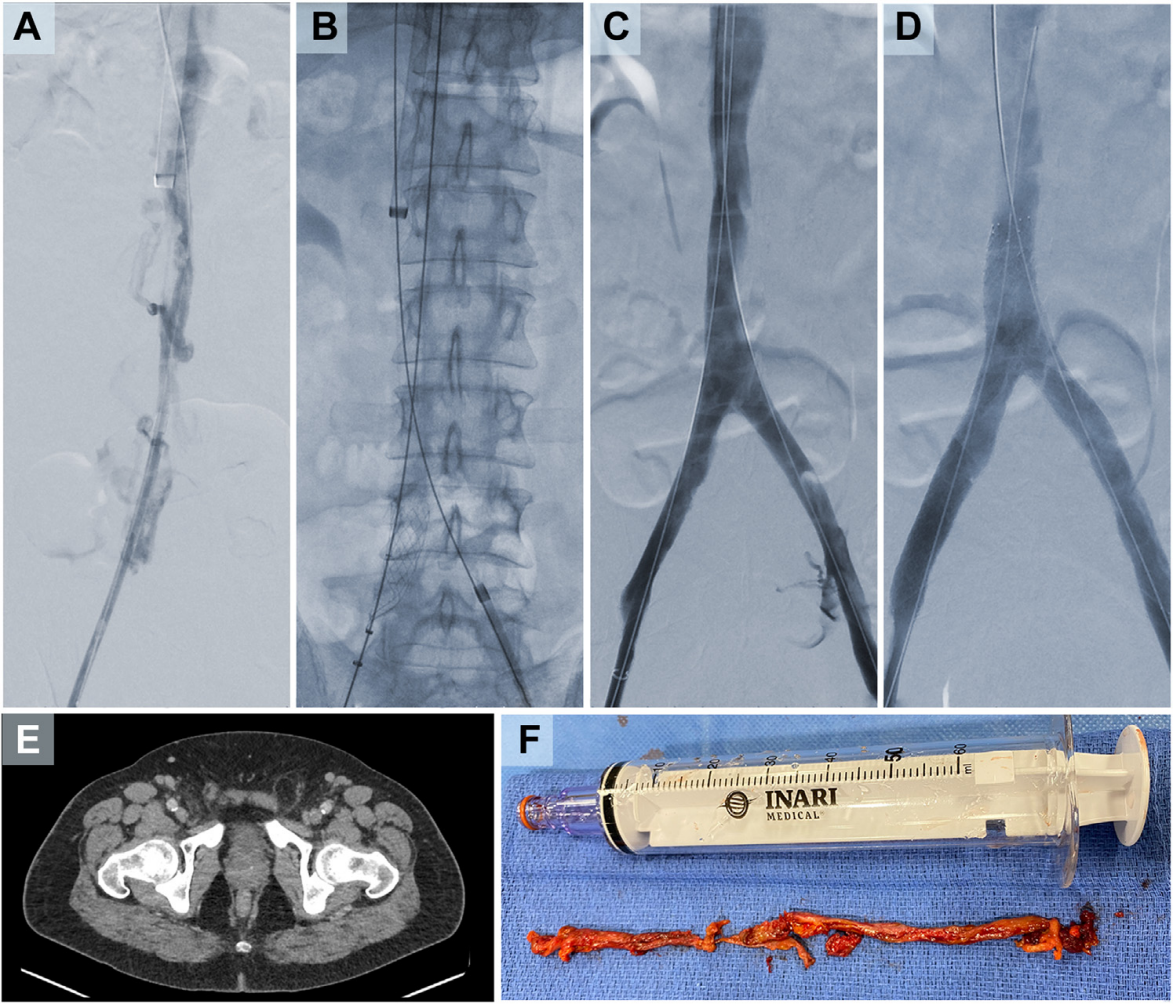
A, Venogram demonstrating chronic occlusion below the inferior vena cava (IVC) filter. B, After serial dilation and sheath placement, a ClotTriever BOLD catheter was advanced beyond the thrombus and expanded into the vessel. C, Venogram after thrombectomy confirming restored patency of the IVC. D, Digital subtraction angiography showing restored patency and flow to the bilateral common iliac veins (CIVs) and IVC. E, Computed tomography (CT) scan performed after the procedure demonstrating patent bilateral common femoral veins (CFVs). F, Chronic-appearing thrombus extracted from the patient.
